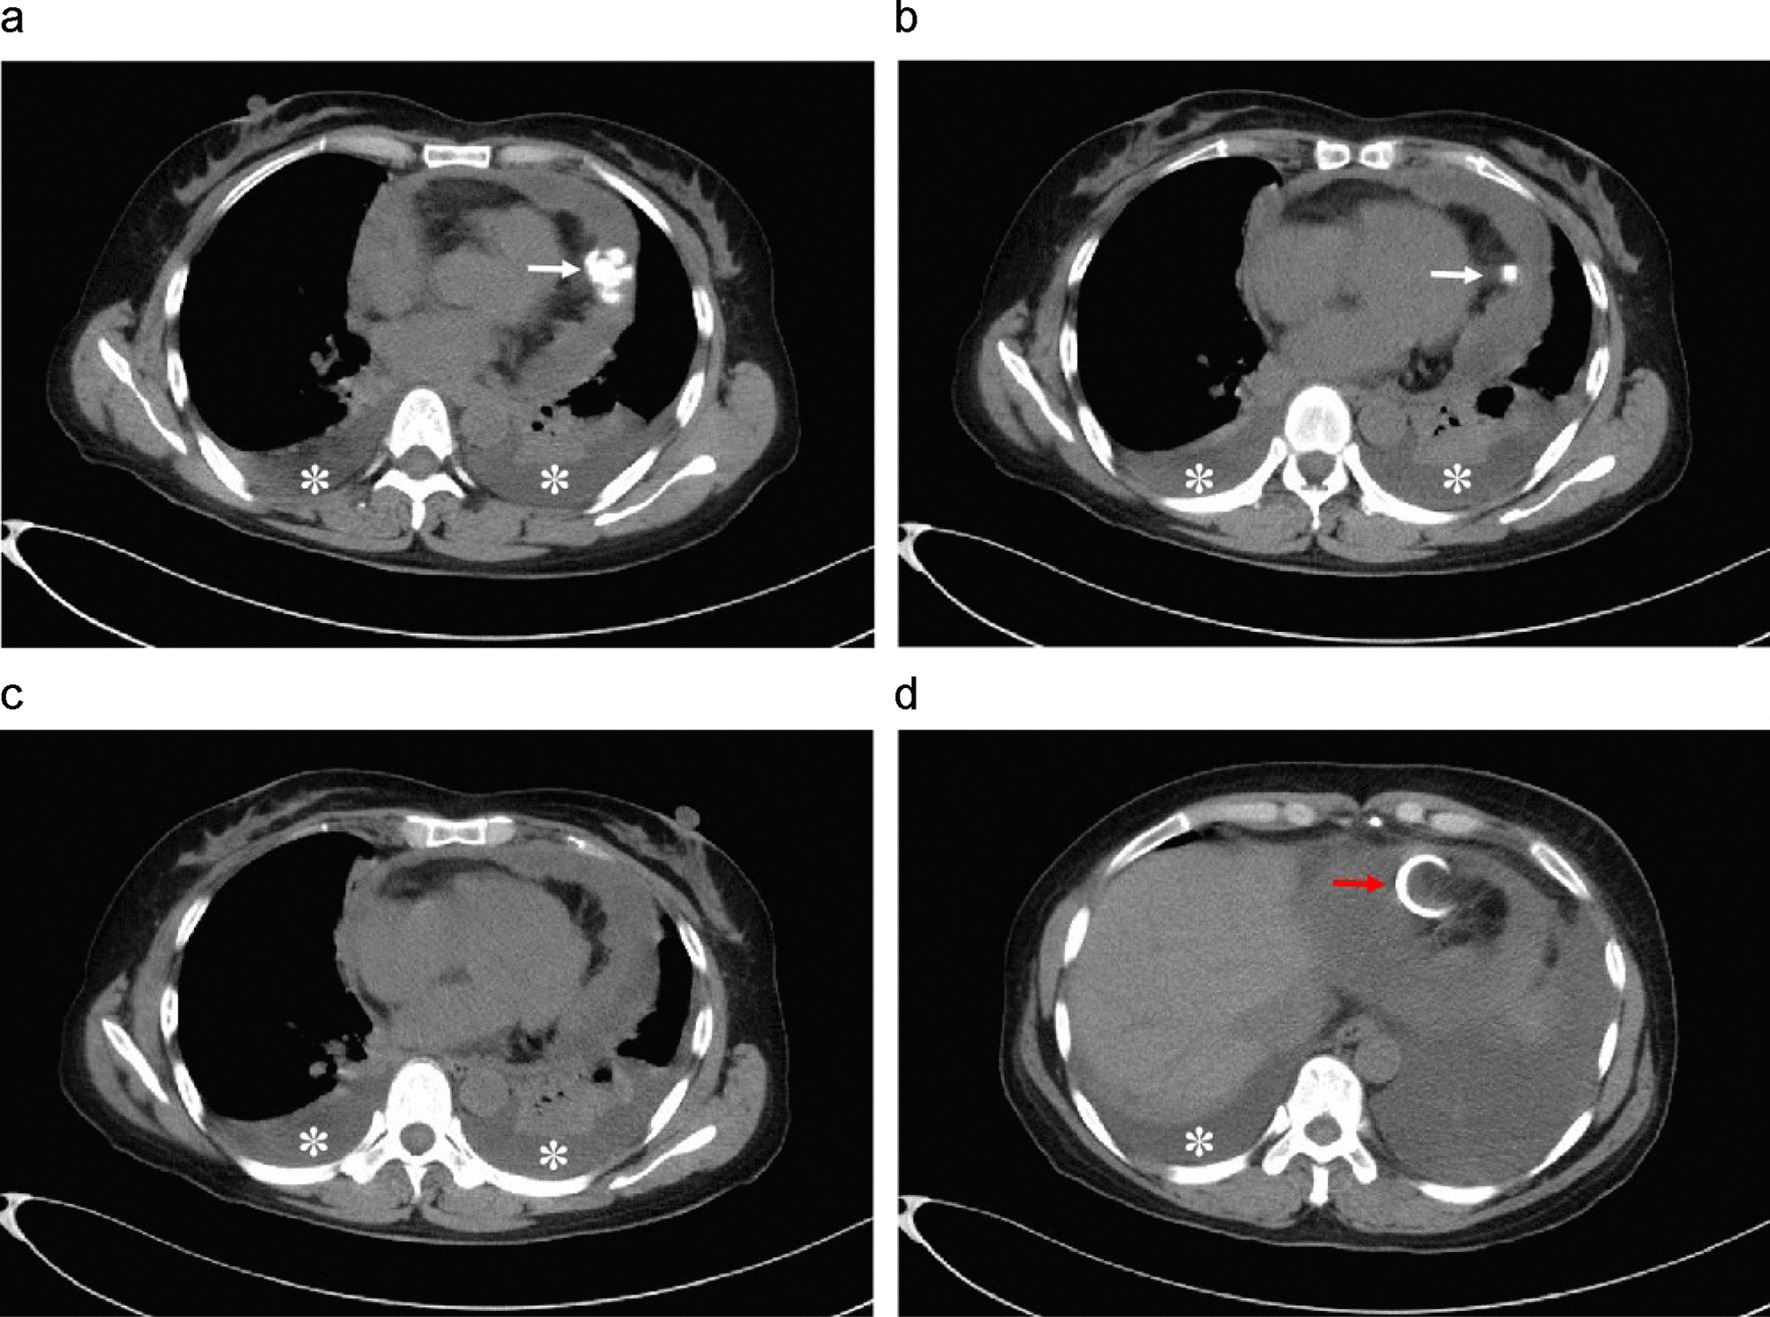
Chest computed tomography (CT). Chest CT scans (soft-tissue window, axial plane) revealing diffuse thickening of the pericardium, more prominent along the left lateral wall with notable calcified foci (A, B white arrow), bilateral pleural effusion (B, C stellate pattern), and an 8 F “pigtail” perforated pericardial drainage catheter (D red arrow)
